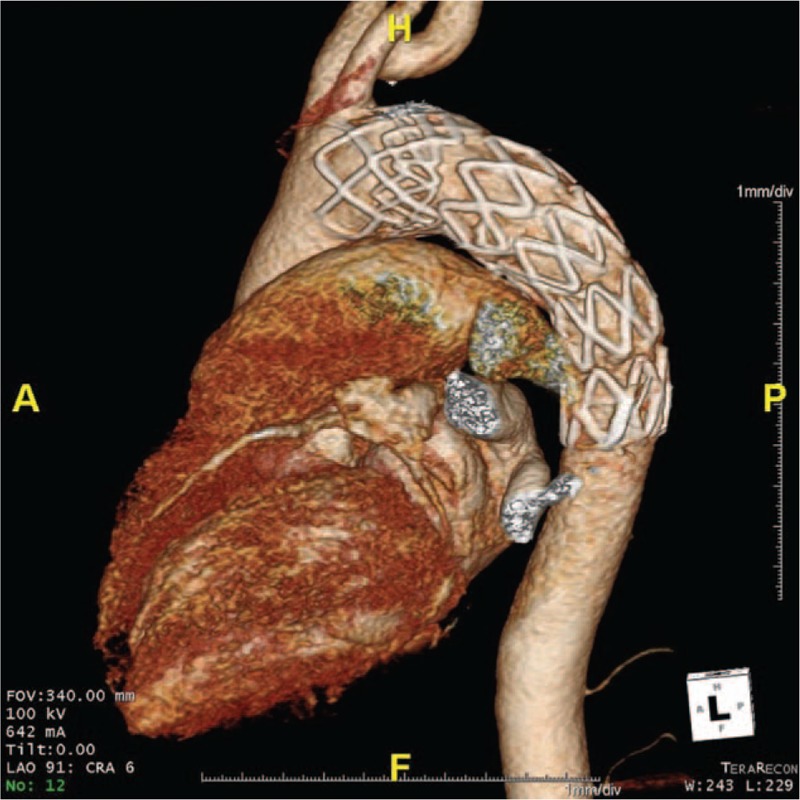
Postoperative three-dimensional computed tomography image of the aorta showing successful deployment of the stent.
